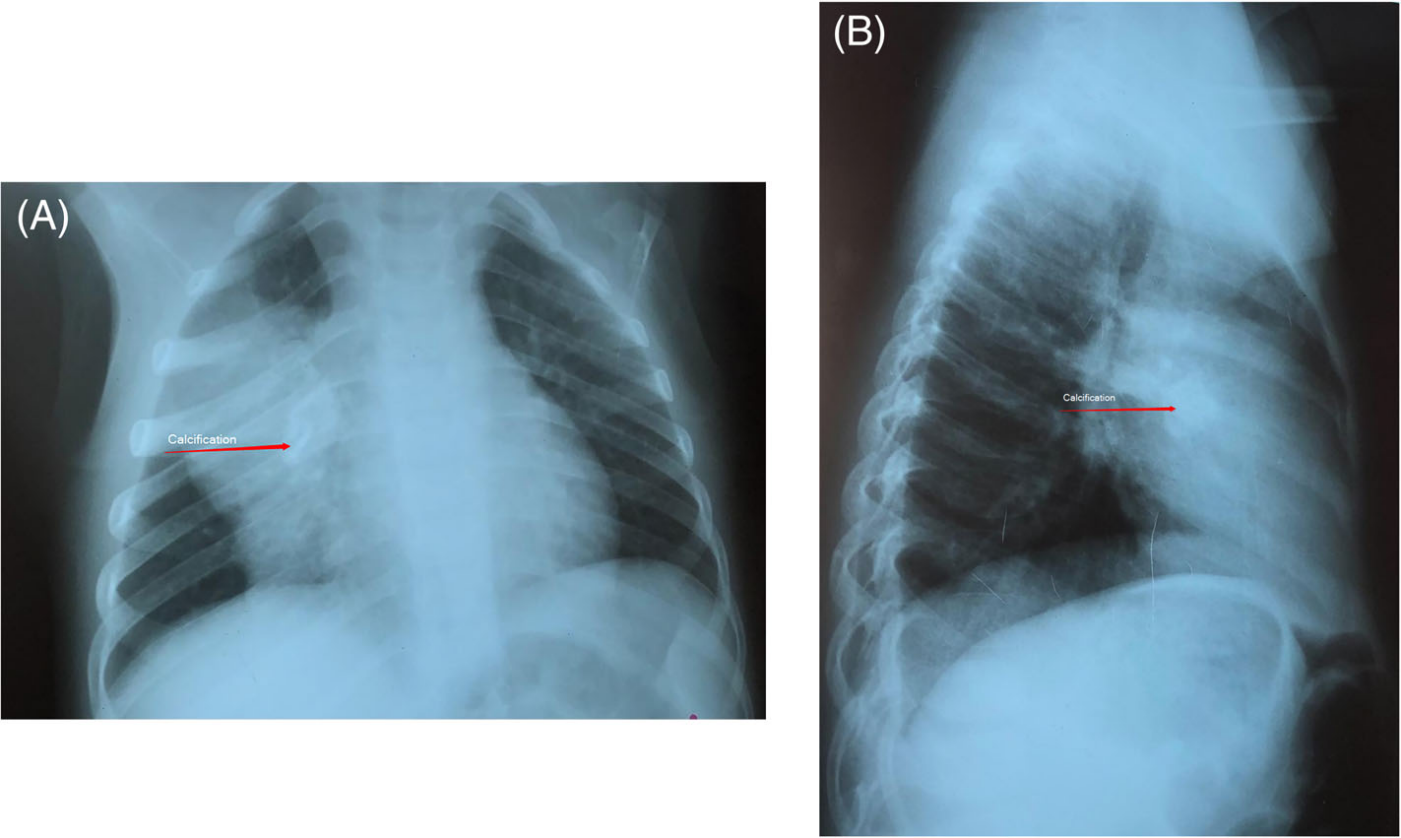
(A) Thorax radiography of the myofibroblastic tumour. (B) Lateral radiography of thorax shows myofibroblastic tumour of the lung
